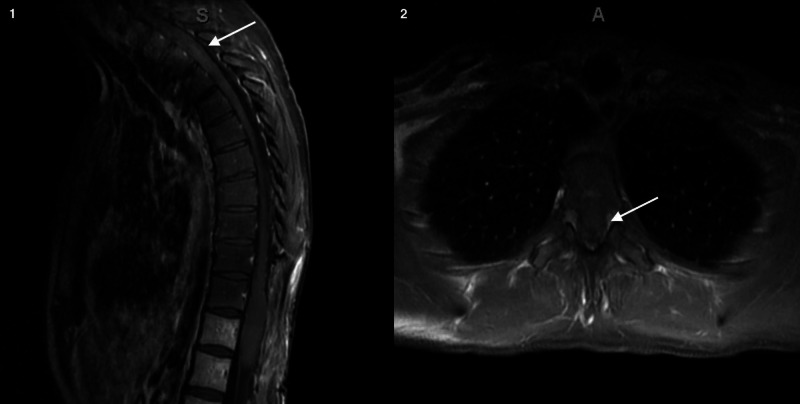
Sagittal (1) and axial (2) T1 MRI with contrast of T1-T5 demonstrating a new expansile cord lesion with heterogeneous signal within the thoracic spinal cord Abbreviations: S, Superior; I, inferior; L, Left; R, Right; A, Anterior.
